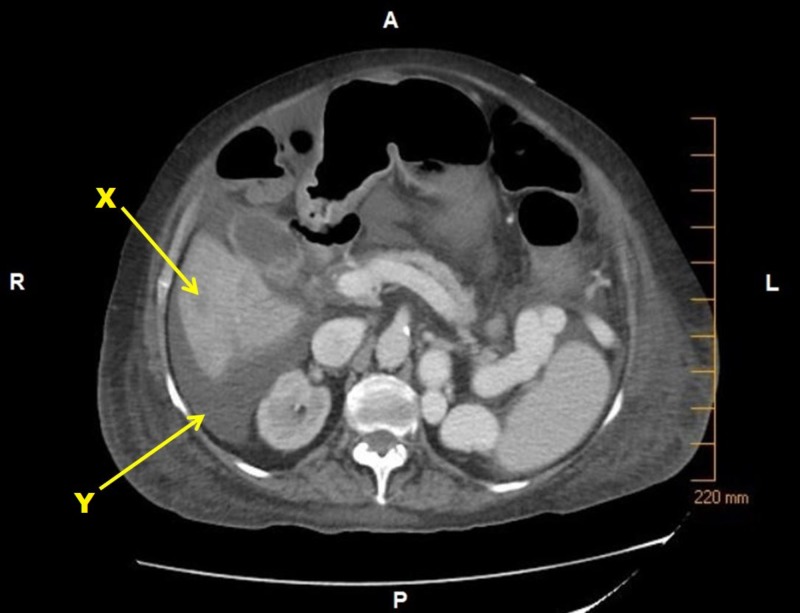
Computed Tomography (CT) Abdomen Computed tomography (CT) abdomen with contrast post-treatment with first-line paclitaxel showing X. Ill-defined low-attenuating lesion in the inferior right hepatic lobe laterally measuring up to 2.0 x 2.1 cm concerning for metastasis Y. Ascites. Legend: A - anterior; P - posterior; R - right; L - left
